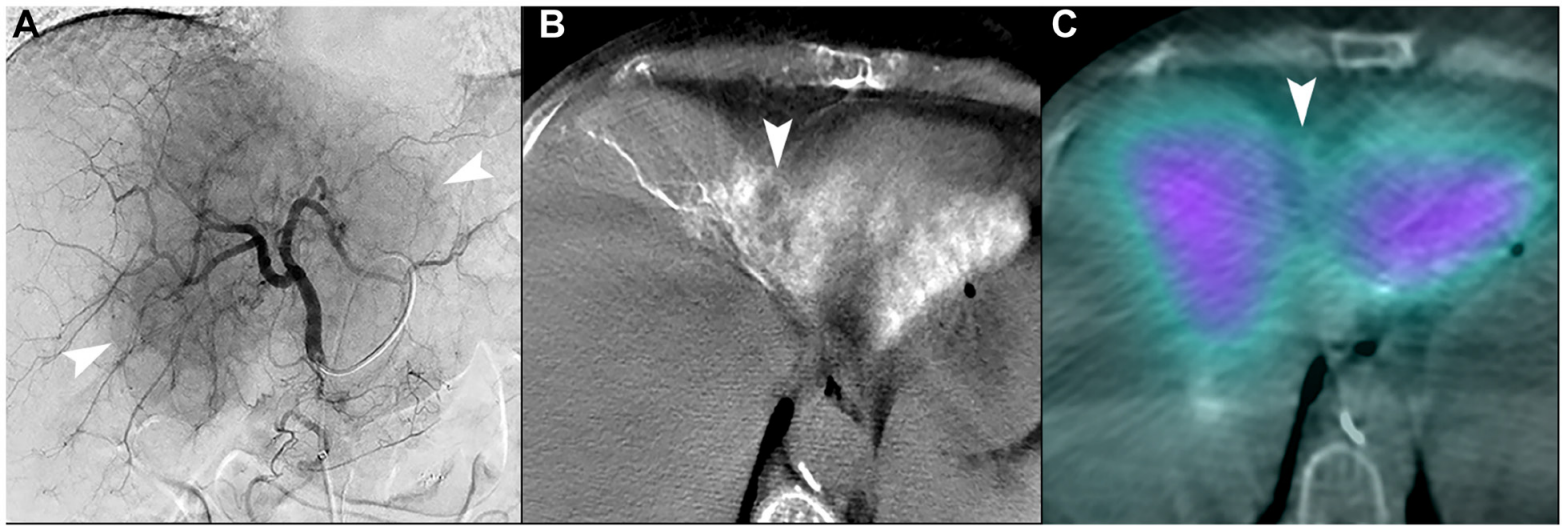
Unfavorable macrovascular and microvascular arterial conduit in two patients with intrahepatic cholangiocarcinoma. (A) Single image from proper hepatic arteriogram shows a large hypervascular iCCA (arrowheads). Cone-beam computed tomography of multiple branches (not shown) demonstrated that tumor blood supply would require treatment of non-expendable liver, which could not be adequately attenuated with distal angiosomal truncation representing unfavorable macrovascular conduit. Ablative intent radioembolization was not offered. (B) Cone-beam computed tomography of a different patient shows an iCCA in segment IVA with poor enhancement of tumor (arrowhead). (C) Bremsstrahlung SPECT/CT after radioembolization showed poor uptake of microspheres in the tumor (arrowhead), representing an unfavorable microvascular conduit.
